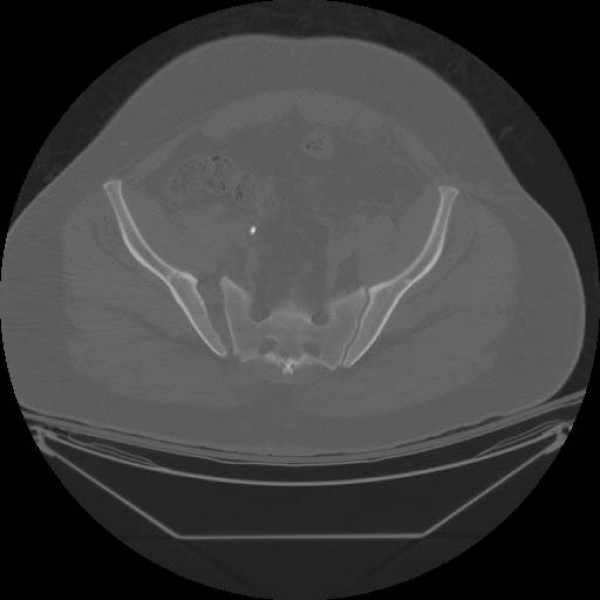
Presenting representative axial pelvic CT cut showing complete right sacroiliac joint disruption.
